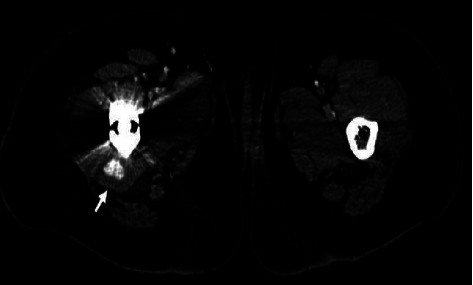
CT with contrast showing the right deep femoral artery pseudoaneurysm.
